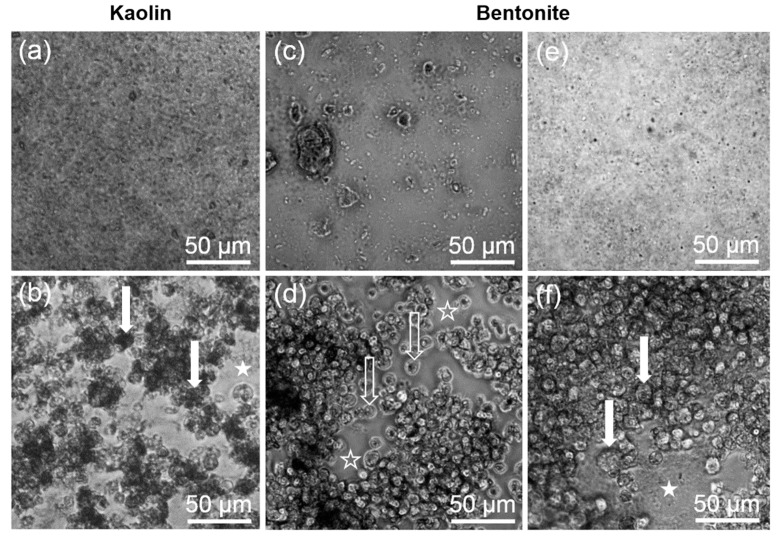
Gravitationally settled kaolin and bentonite particles, and uptake by NR8383 cells after 16 h. (a,c,e) Settled particles in cell-free control wells. (b,d,f) NR8383 cells incubated for 16 h with the same particles as shown above. (a,b) Kaolin (180 µg/mL) after ultrasonic dispersion for 10 s. (c,d) Bentonite (180 µg/mL) after ultrasonic dispersion for 10 s. (e,f) Bentonite (180 µg/mL) after ultrasonic dispersion for 300 s. Filled arrows point to dark, particle-filled cells (b), or deteriorated cells with granular appearance (f). Filled asterisks mark areas where settled, non-ingested particle agglomerates are visible (b,f). Open arrows (d) show largely uncompromised cells; open asterisks (d) mark areas that had been cleared from particulate matter by phagocytosing cells.
